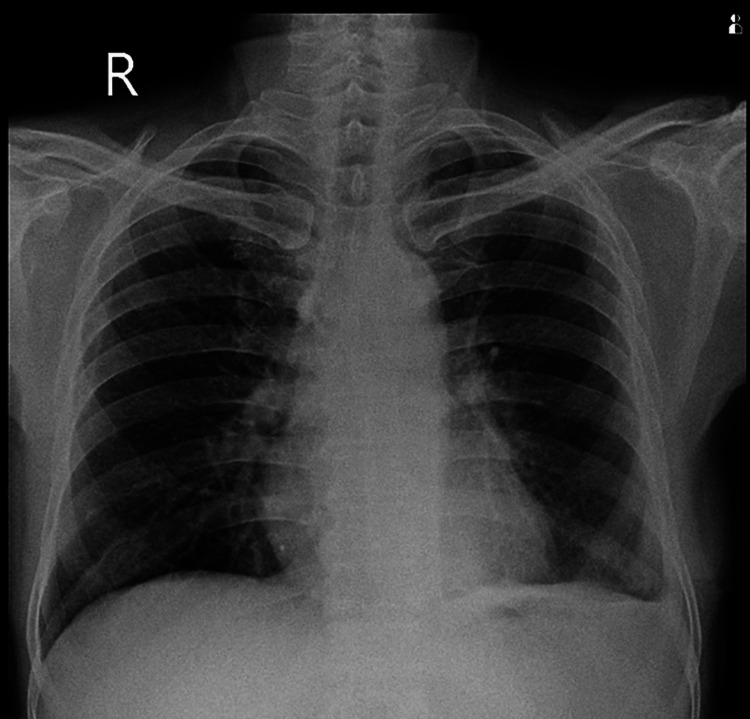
Chest X-ray post-operative day 45
